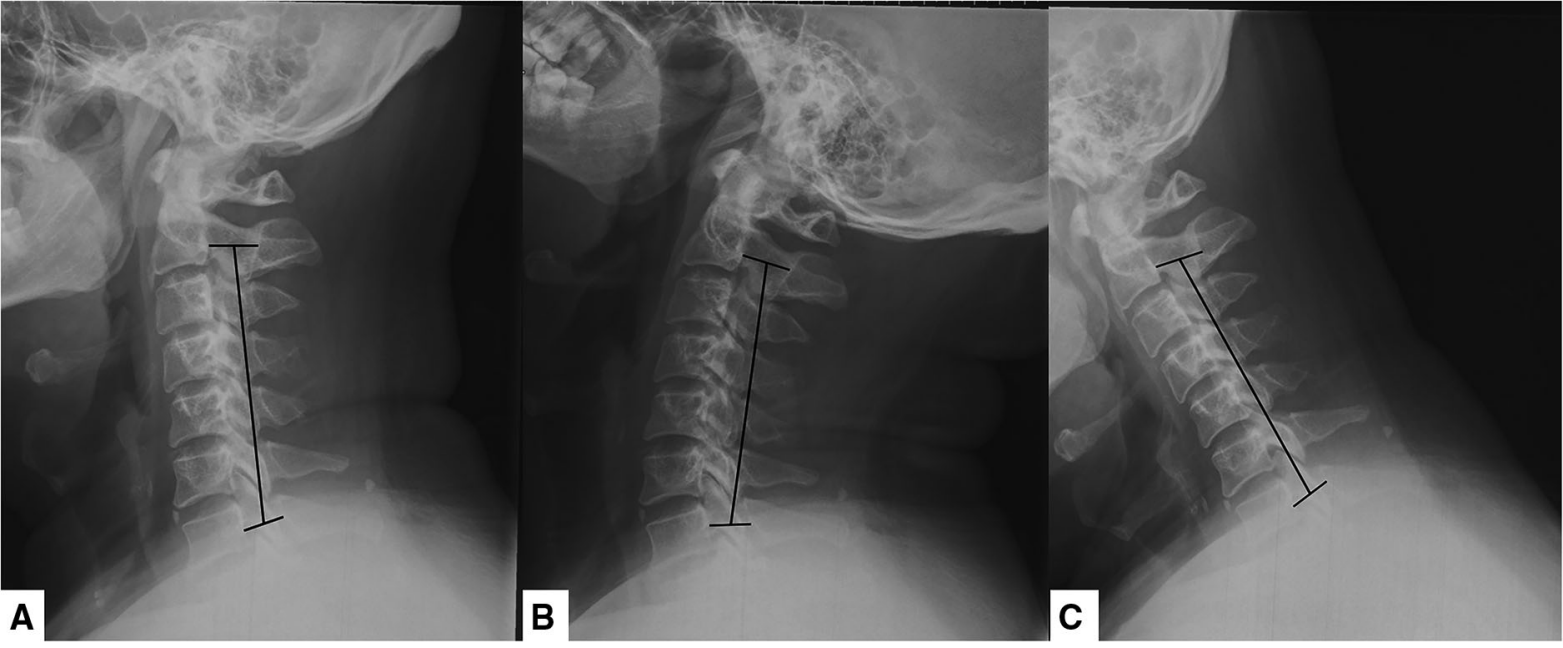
Cervical radiographs in different dynamic position. (A) Neck-neutral position; (B) neck-flexed position; (C) neck-extended position.
